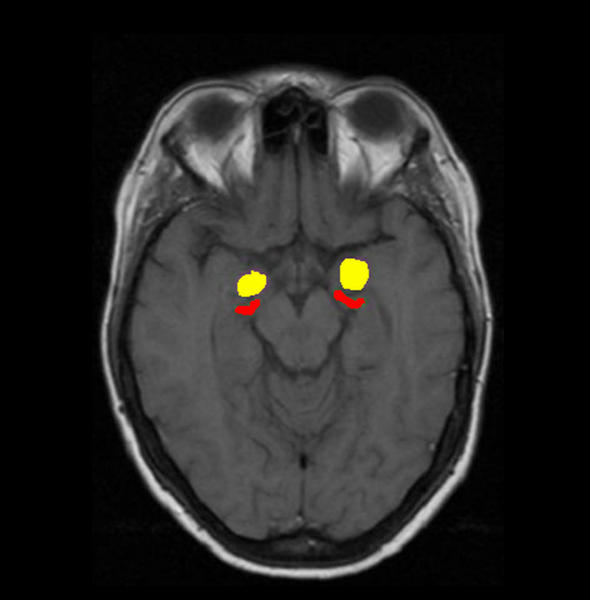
Axial T1 Weighted Anatomical MRI Scan Showing Segmentation of the Amygdala (Yellow) and the Hippocampus (Red)
